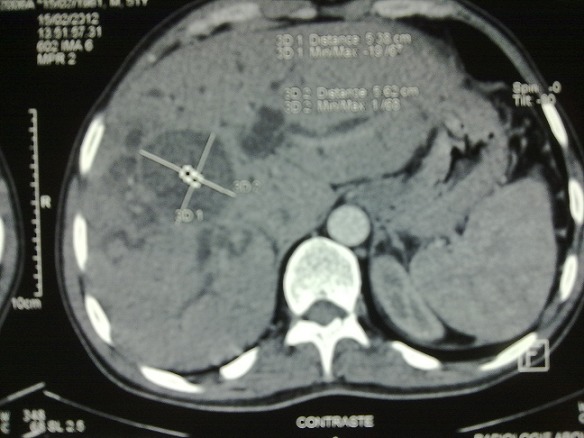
Abdominal CT showing hydatid cyst of 4th & 5th segments of liver communicating wit dilated CBD
